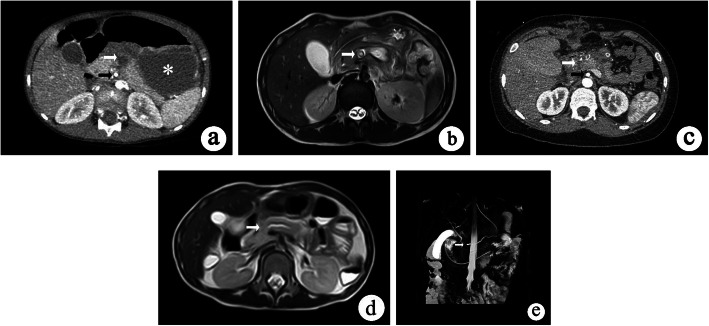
Typical imaging results indicated by abdominal CE-CT and MRI examinations in children who sustained grade III and IV injuries (white arrow: transection or parenchymal injury with duct injury; black arrow: superior mesenteric artery; asterisk: pancreatic pseudocysts). a-b CE-CT and MRI, axial scan: grade III pancreatic injury, with lesion in the distal part of the duct on the left side of superior mesenteric artery or the spine. c-d CE-CT and MRI, axial scan: grade IV pancreatic injury, with lesion in the proximal part of the duct on the right side of superior mesenteric artery or the spine. e MRCP: grade IV pancreatic injury, with lesion in the proximal part of the duct on the right side of the spine
